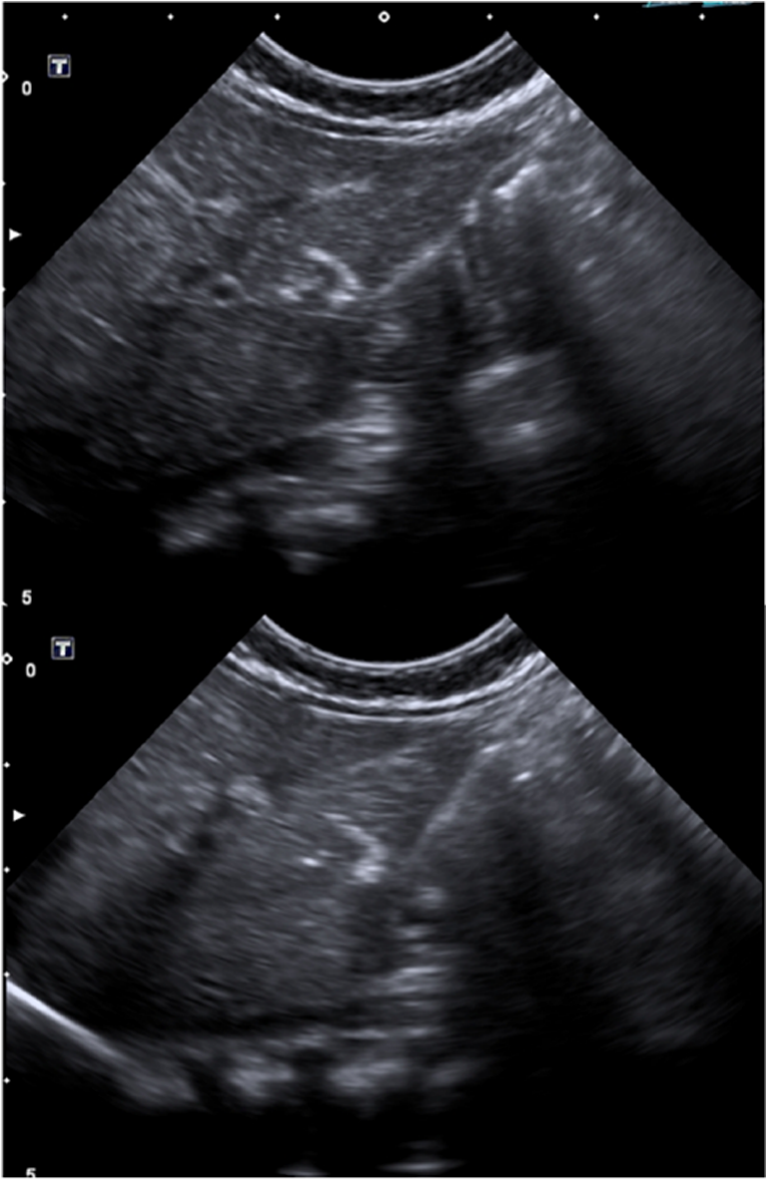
Ultrasound-guided percutaneous intrahepatic injection of human liver stem cells (HLSCs) in an infant with neonatal-onset inherited hyperammonemia while in stable metabolic conditions. HLSCs injection and real-time ultrasound images are presented
